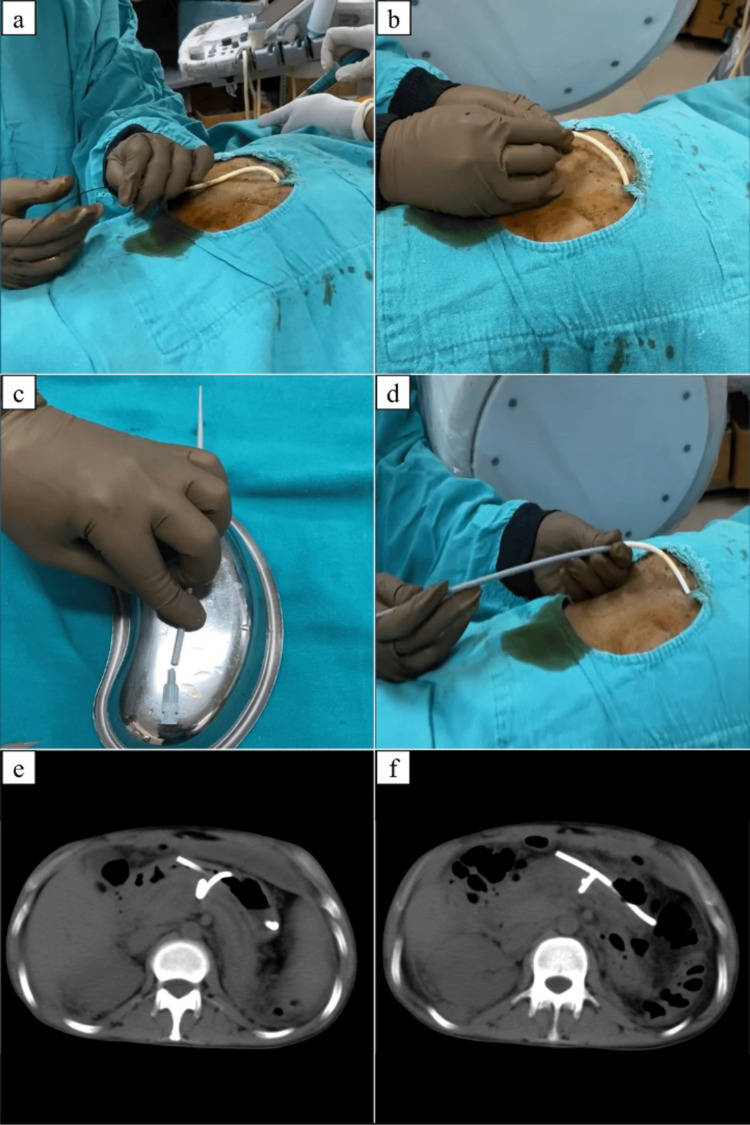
Steps of internalization of transgastric drain The stomach is inflated with air before the procedure. (a) Terumo guidewire was inserted through the drain into the collection. (b) One end of the drain and its two wires are cut, while the Terumo guidewire is still in place and the cut end is removed. (c) One end of Coon’s dilator is cut. (d) The cut end of Coon’s dilator is used to push the drain inside the stomach. (e and f) CT images showing the final position of the internalized drain within the stomach after the removal of the guidewire and dilator
